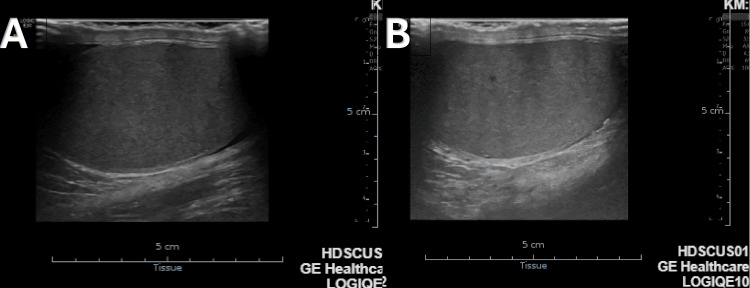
Testicular ultrasound A: right testicle; B: left testicle. Right and left testicle volume 16 cubic centimeter (cm^3^).
